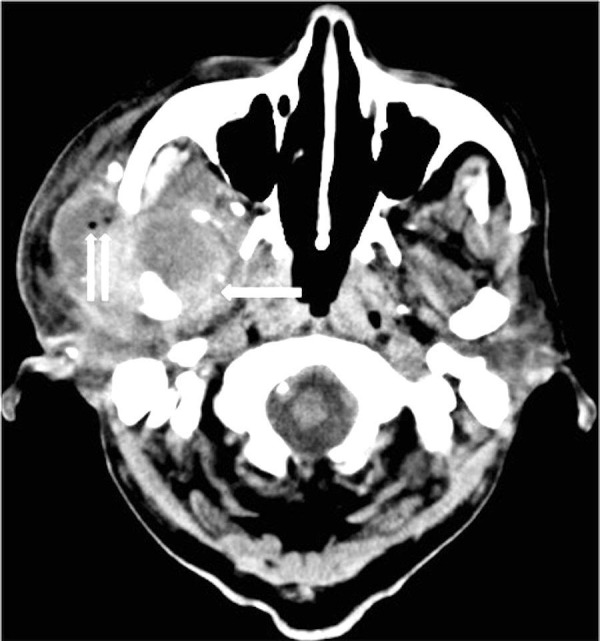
Noncontrast head computed tomography demonstrates heterogeneous mass in the right masticator space (arrow). Note the locules of air just lateral to the zygomatic arch (double arrow).
